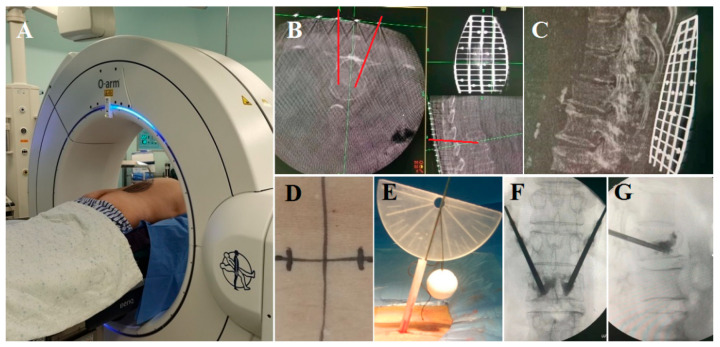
Intraoperative images of a 68-year-old woman with an L1 vertebral compression fracture. (A–C) Three-dimensional CT scans carried out using the O-arm were used to perform preoperative planning. (D) The entry point on the skin was determined using the body surface locator before the operation. (E) A 3D-printed guide device was used to determine the bone puncture point and insertion angle of the trajectory during the operation. (F,G) Cement was injected into the vertebra through bilateral PKP.
